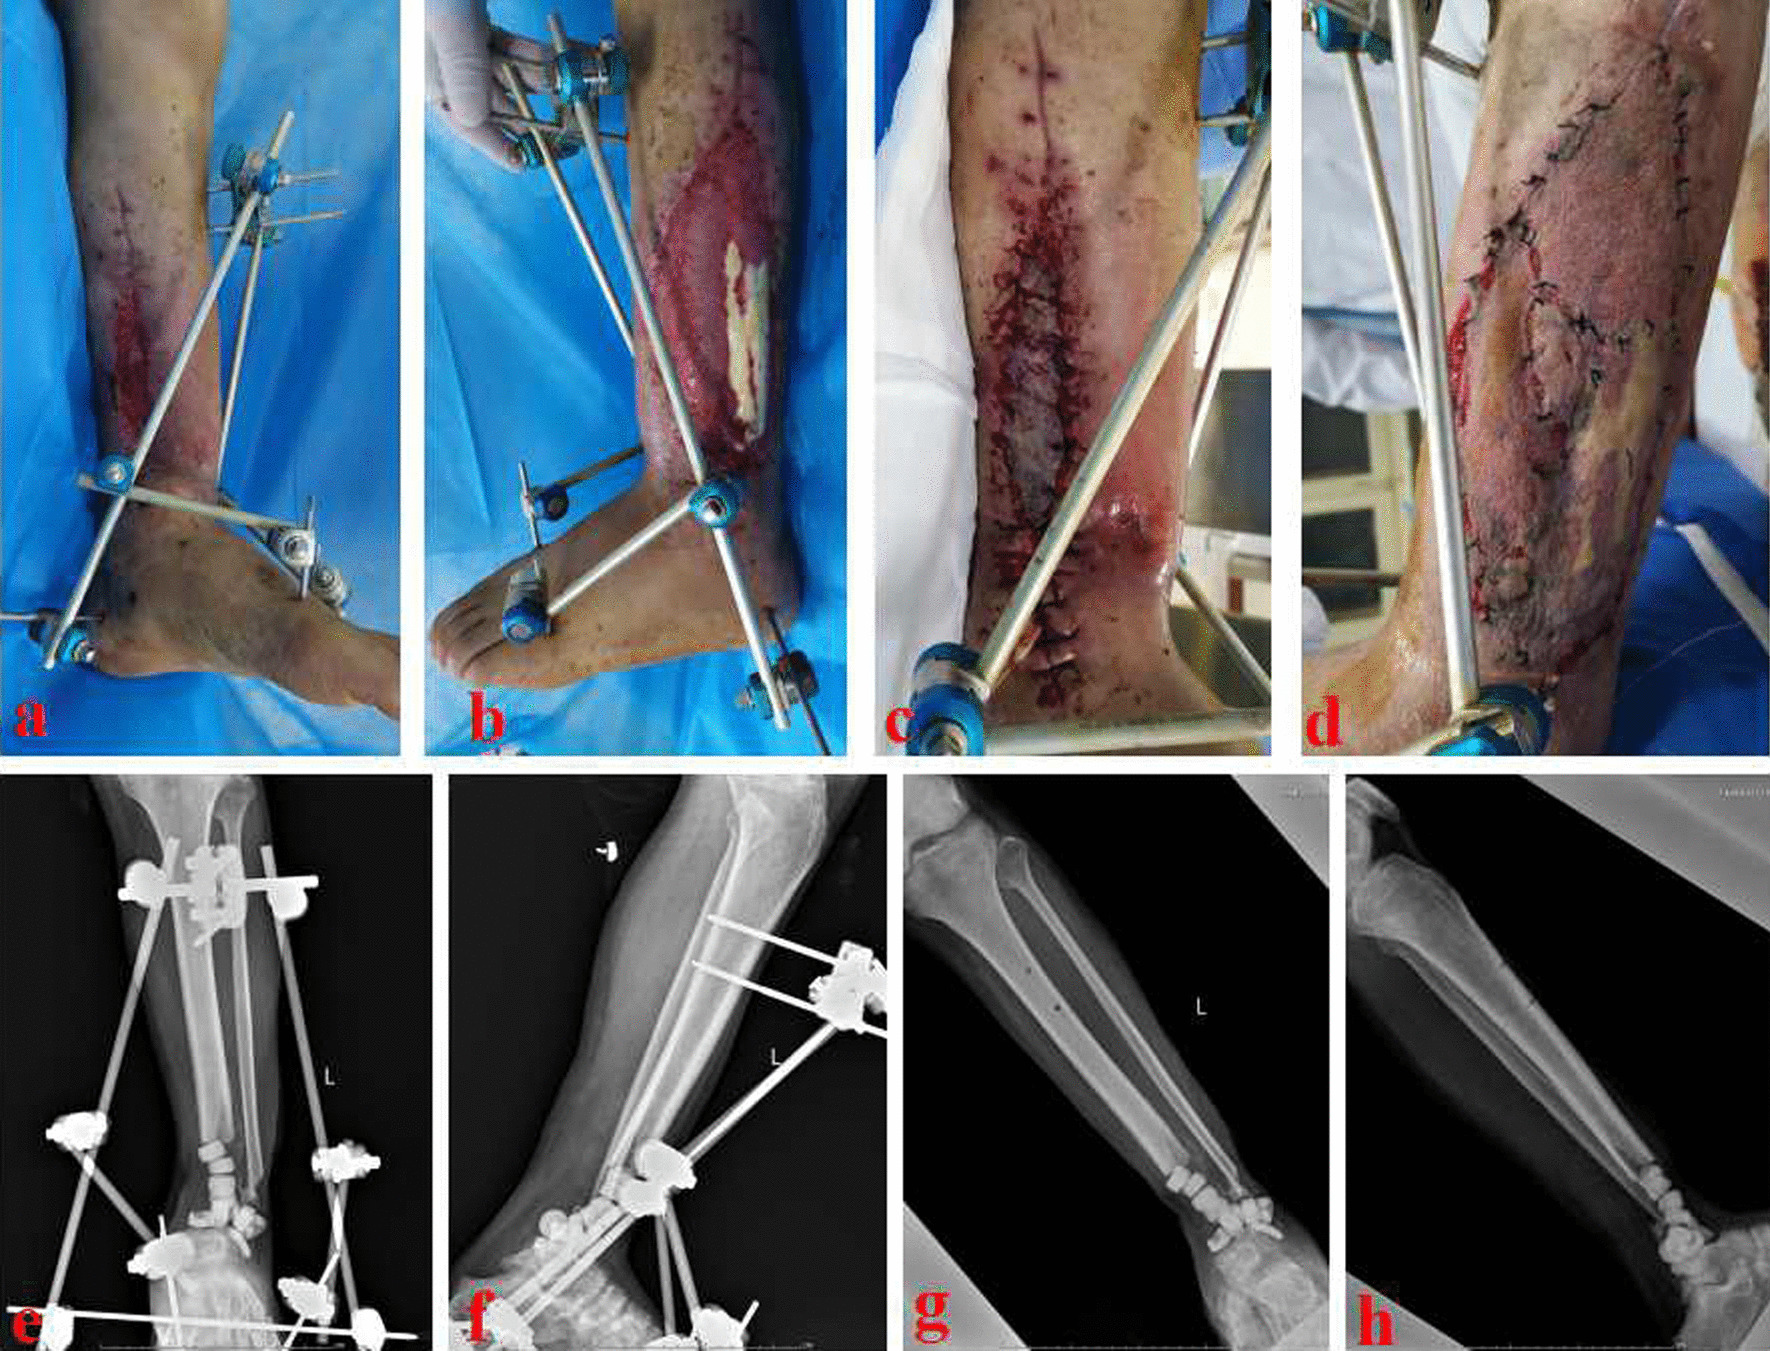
The patient underwent debridement, bone cement filling and soft tissue reconstruction. a and b After debridement, there was still a large area of skin and soft tissue defects; c and d The skin and soft tissue defects were repaired by skin grafting; e and f: X-ray films after bone cement filling; g and h X-ray films after removing the temporary external fixator
